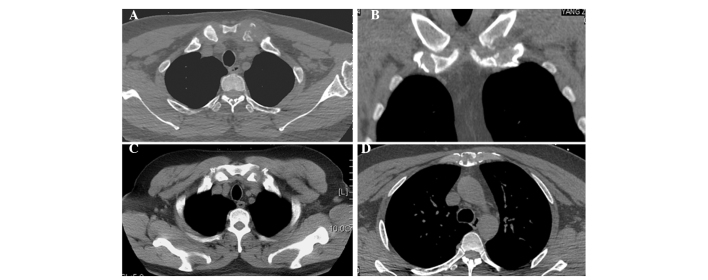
Computerized tomography (CT) scans of the sternum. The sternoclavicular joints revealed osseous erosions on (A–C) the left sternoclavicular joint area and (D) the manubrium.
